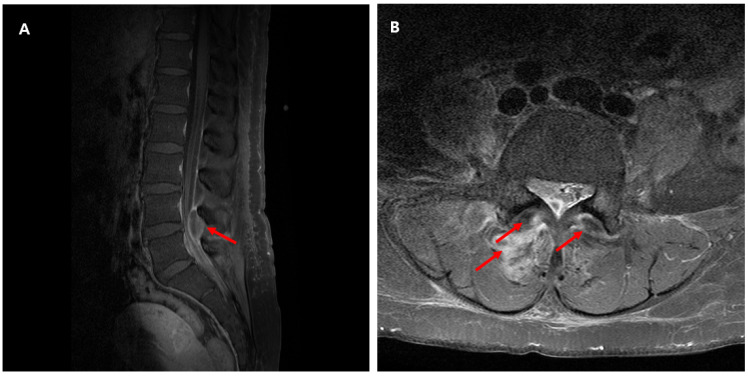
Lumbar spine magnetic resonance imaging (MRI). (A) Contrast-enhanced T1-weighted MRI Dixon water (B) T1-weighted MRI SPIR coronal. (A) Lumbar spine MRI showed epidural abscess (approximately 0.6 × 1.2 × 2.7 cm) at the right posterio-lateral aspect of the lumbar spine at the lumbar 4−5 level. (B) Infectious arthritis with capsular rupture of lumbar 4−5 level and lumbar 5-sacral 1 level of facet joints with periarticular abscess formation.
